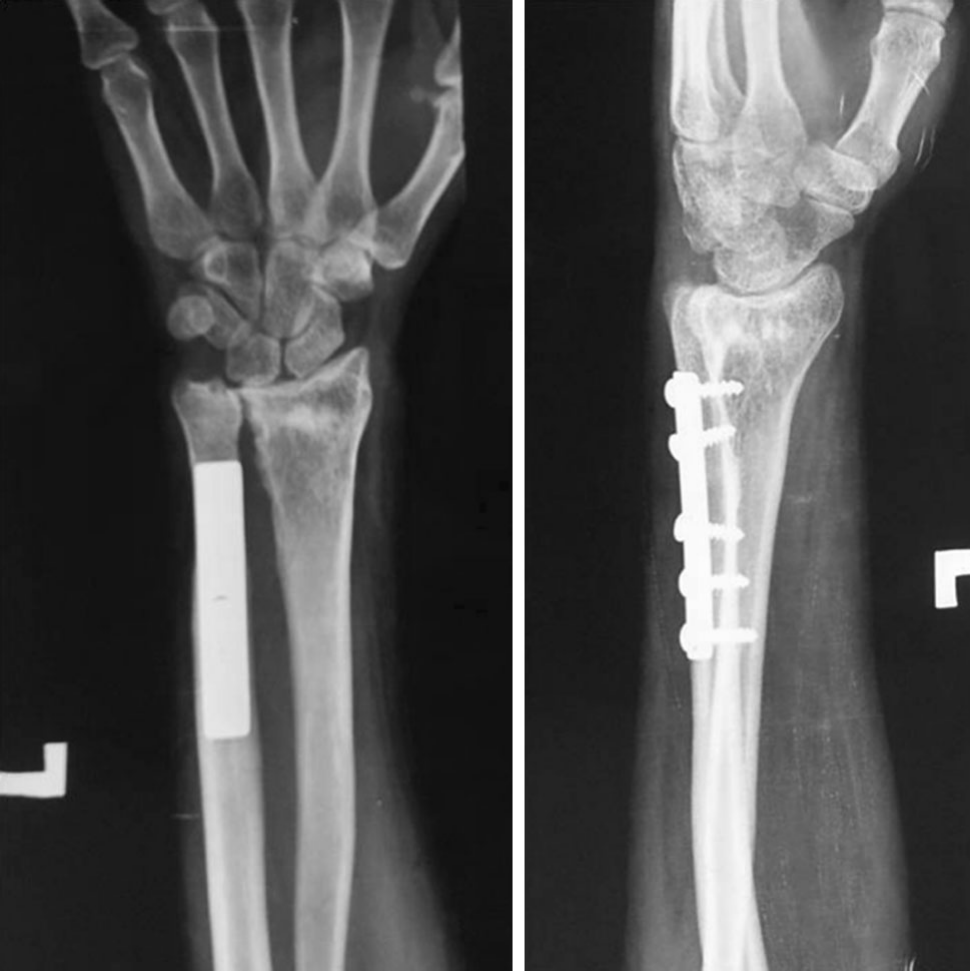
Radiographs at final follow-up at 2 years
